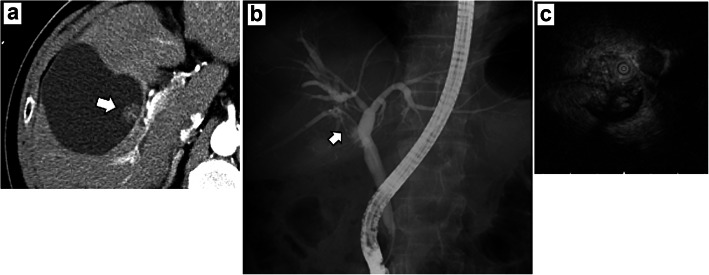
Imaging findings from the liver recurrence. a Dynamic computed tomography revealed an enhanced tumor within a cystic substance during the arterial phase (white arrow). b Endoscopic retrograde cholangiography revealed a filling defect (white arrow). c Intraductal ultrasonography showed a papillary tumor in the dilatation of right hepatic duct
